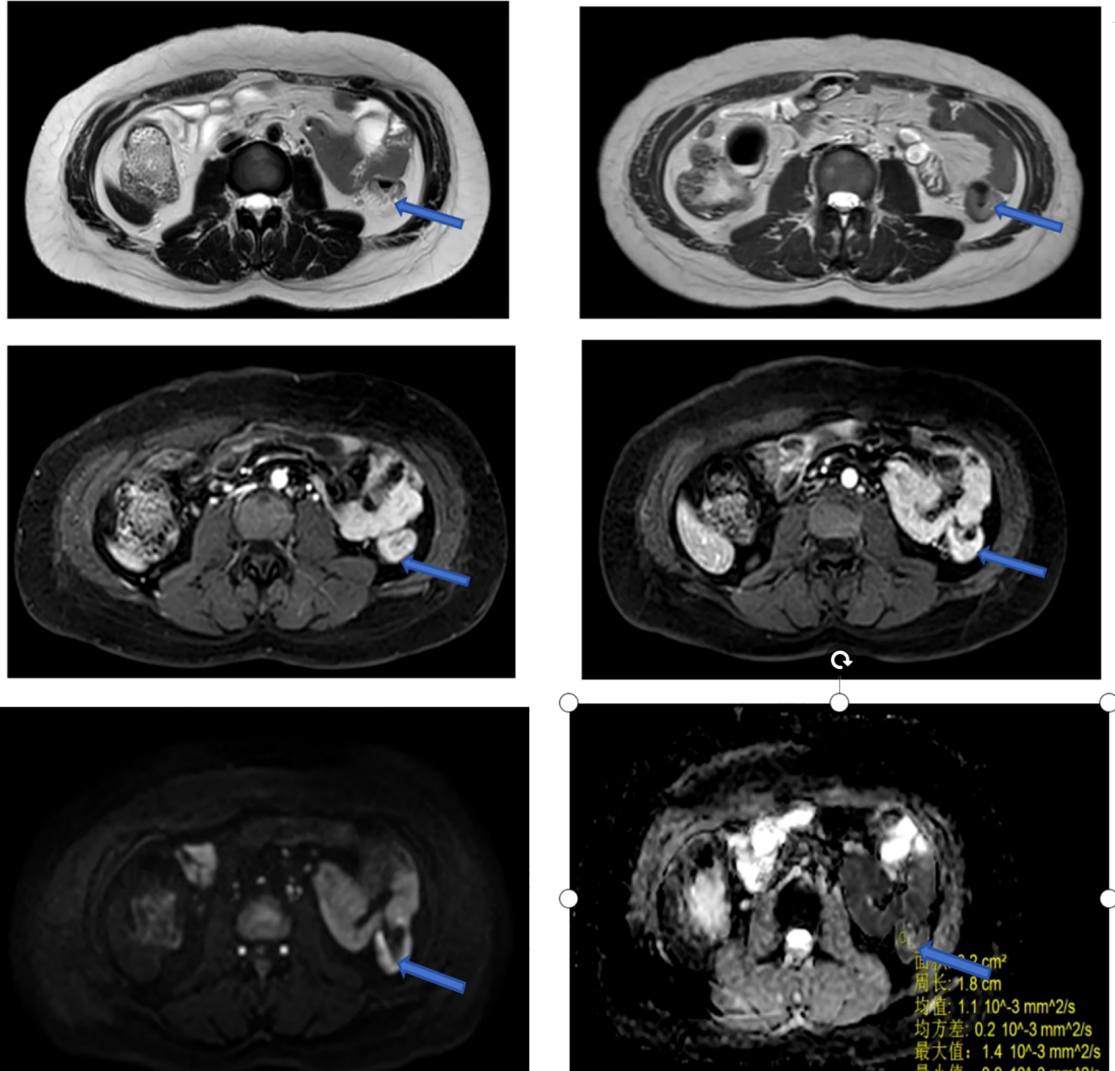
Images from the first MRI exam on Feb 24, 2024, with blue arrows marking the tumor sites.
